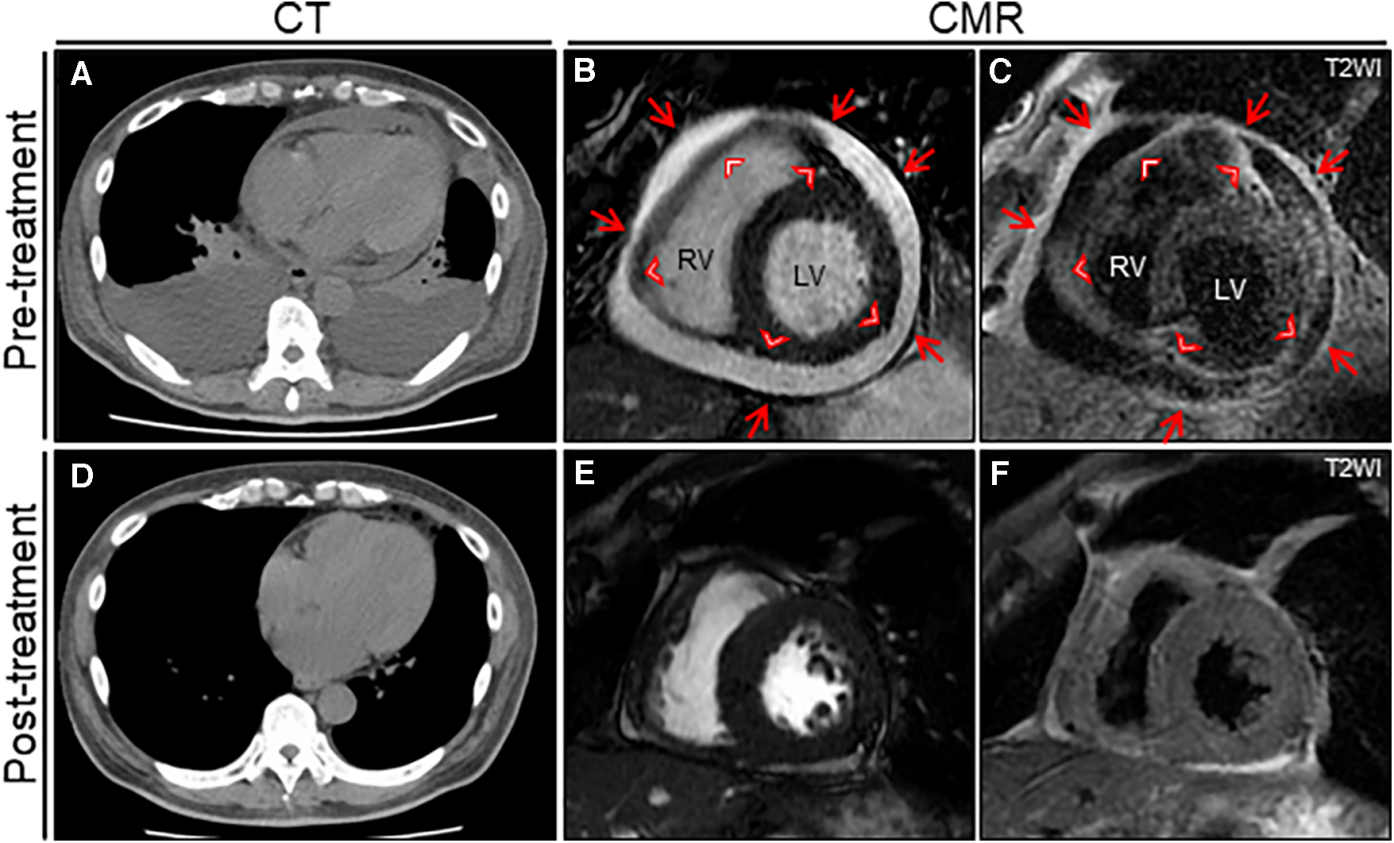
The treatment effect on chest computed tomography (CT) and cardiac magnetic resonance (CMR) findings. Chest CT reveals moderate pericardial and bilateral pleural effusions with passive atelectasis (A) that resolves completely at the 6-month follow-up (D) Cine CMR reveals a moderate pericardial effusion at baseline (B) that resolves significantly at the 6-month follow-up (E) Note the entire thickening of epicardium (arrowheads) and pericardium (arrows). T2-weighted image (T2WI) shows active diffuse pericardial edema of the epicardium (arrowheads) and pericardium (arrows) observed at baseline (C) that resolves significantly at the 6-month follow-up (F). LV, left ventricle; RV, right ventricle.
